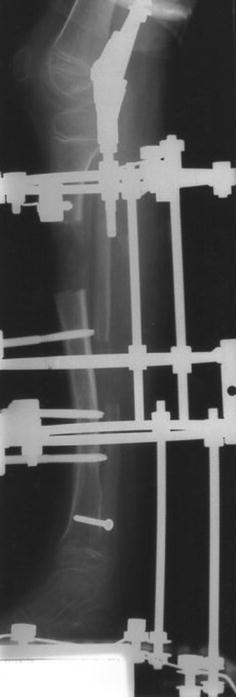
Radiograph lateral view during lengthening
